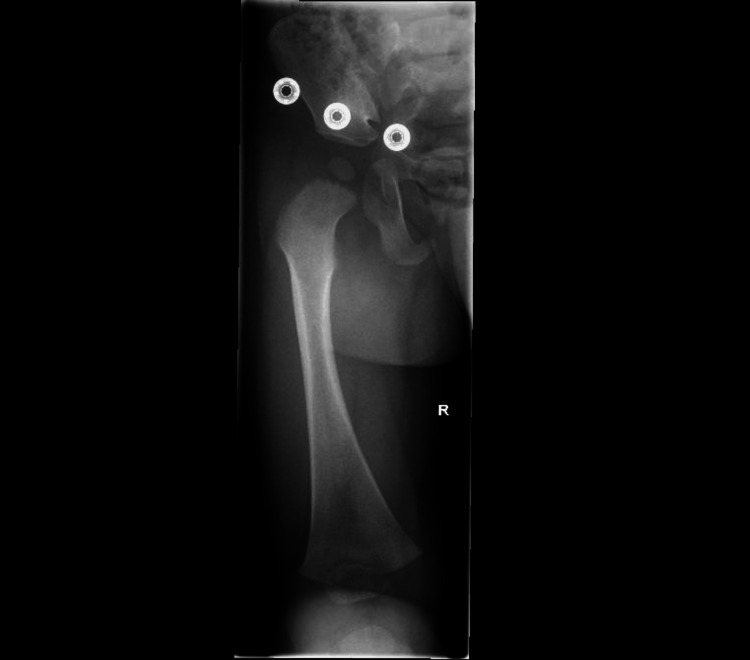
An X-ray of the femur with an anterior-posterior view shows no acute femoral fracture or focal bony lesion. Normal alignment is preserved at the knee joint.
